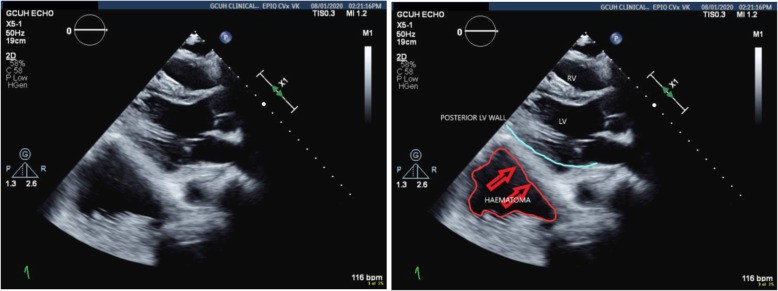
Parasternal long axis view showing the haematoma compressing the posterior wall of the LV
